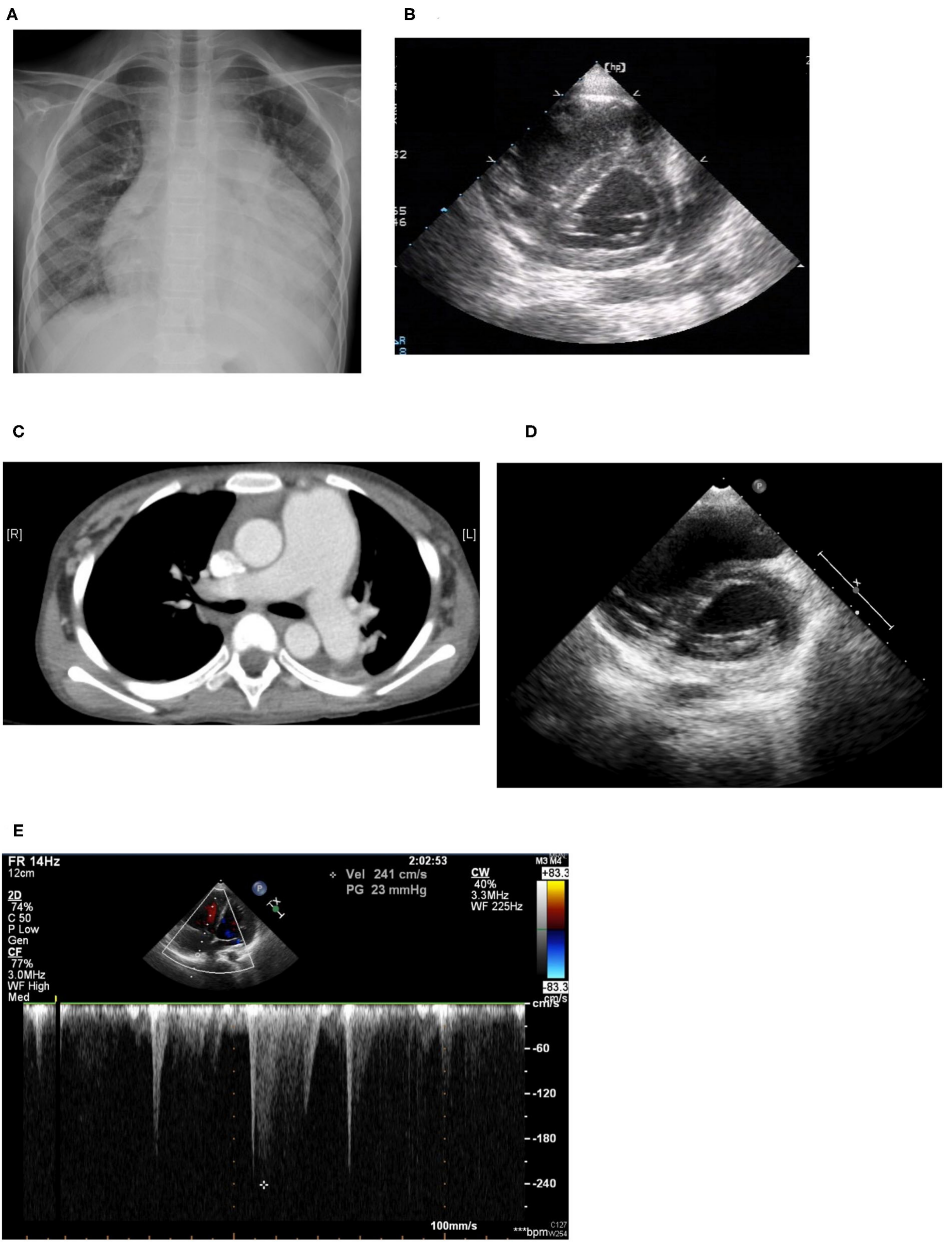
(A) Cardiomegaly and pleural effusion seen on chest x-ray on admission. (B) Echocardiogram on admission: Right ventricle dilatation due to pulmonary hypertension with shift of the intraventricular septum to the left, resulting in a D-shaped left ventricle (D-sign). (C) Chest CT: Dilated pulmonary arteries. (D) Echocardiogram performed 1 year later: improving pulmonary hypertension with resolution of “D-sign.” (E) Echocardiogram performed 1 year later: Improving pulmonary hypertension with tricuspid regurgitation with maximum velocity of 2.41 m/s, estimated right ventricle systolic pressure 30 mmHg.
